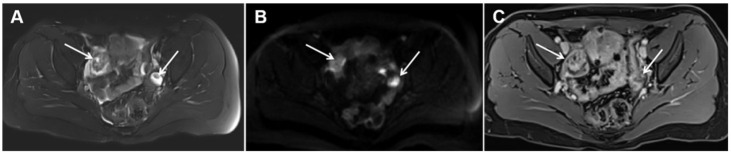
Case 1, a woman with right ILC 2 years after breast cancer surgery, asymptomatic on routine screening. (A) Axial T2WI shows bilateral ovarian cystic-solid masses (arrows); (B) axial DWI (b = 800 s/mm^2^) shows hyperintensity of ovarian masses (arrows); (C) axial contrast-enhanced T1WI shows enhancement of the solid components of the ovarian masses (arrows).
